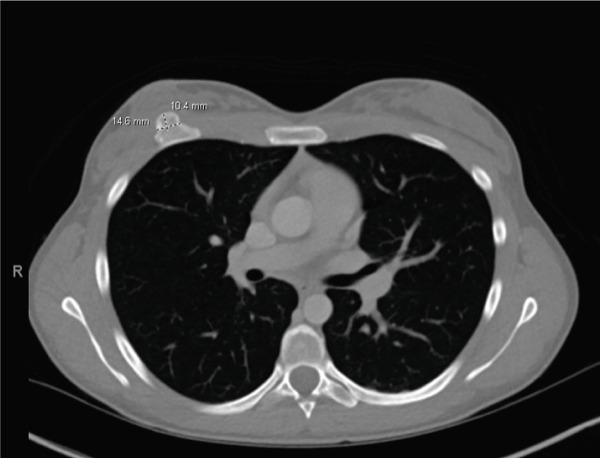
CT with IV contrast identifying bony lesion on the anterior aspect of the right third rib.
